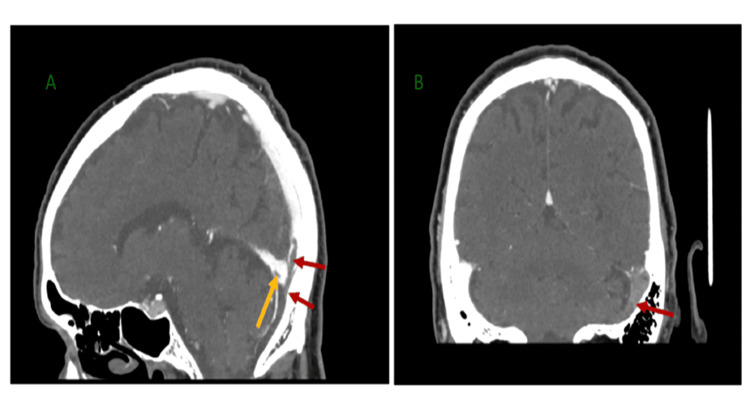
CT venograms. (A) Red arrows indicate a contrast-filling defect/thrombosis in the distal superior sagittal sinus; the yellow arrow indicates a partial filling defect in the straight sinus.
